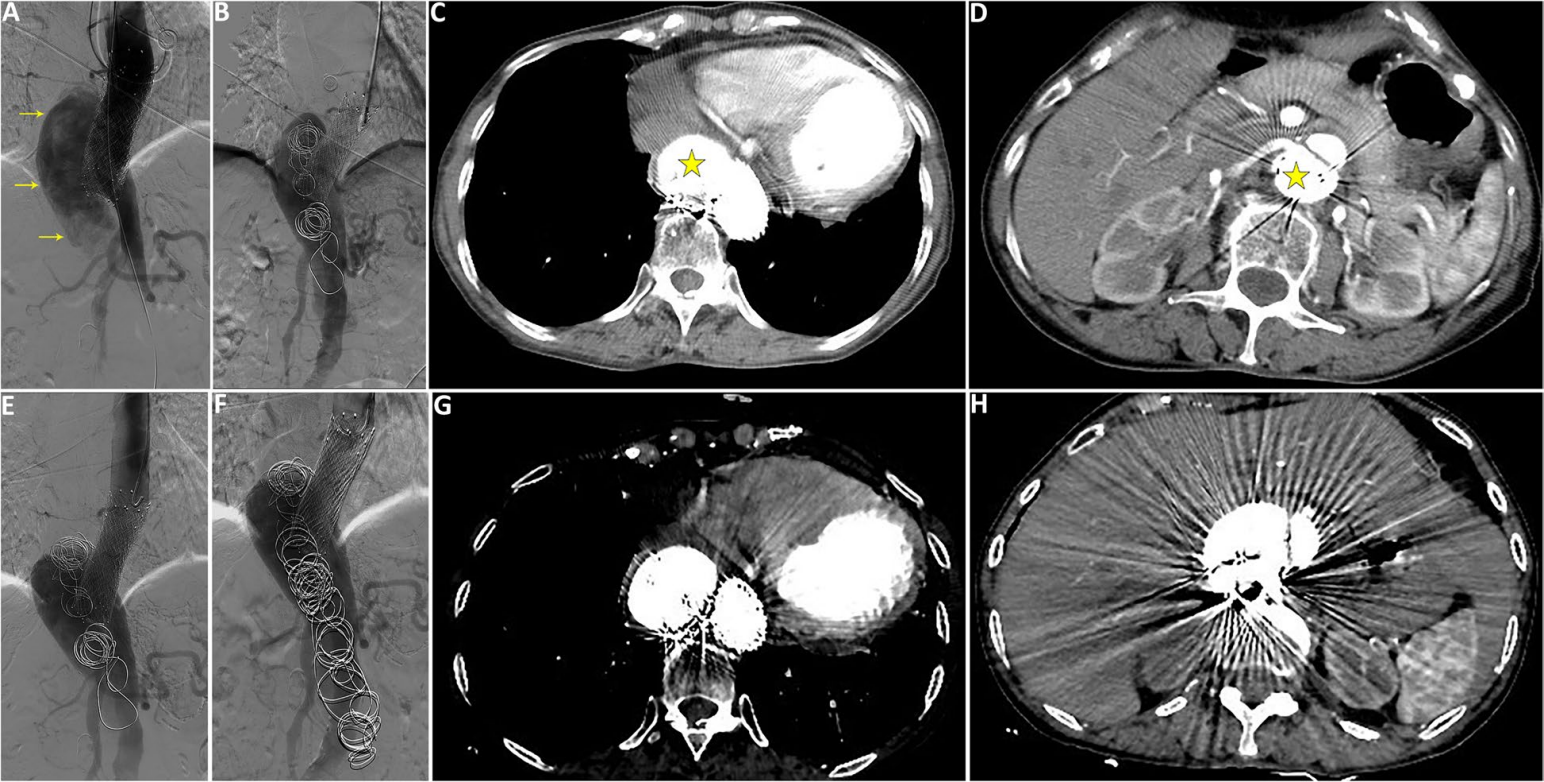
Coil embolization of the false lumen of post-aortic dissection aneurysm. A Preoperative DSA image showing rapid flow velocity in the false lumen (yellow arrow); B Intraoperative image of spring coil embolization of the false cavity, C, D CTA image 1 year after coil embolization showing no obvious thrombogenesis in the false lumen (yellow asterisk). E Preoperative image of the second coil embolization of the false lumen. F Image after the second spring coil embolization of the false cavity. G, H Six months after the second coil embolization, CTA showed still no obvious thrombus in the false lumen
